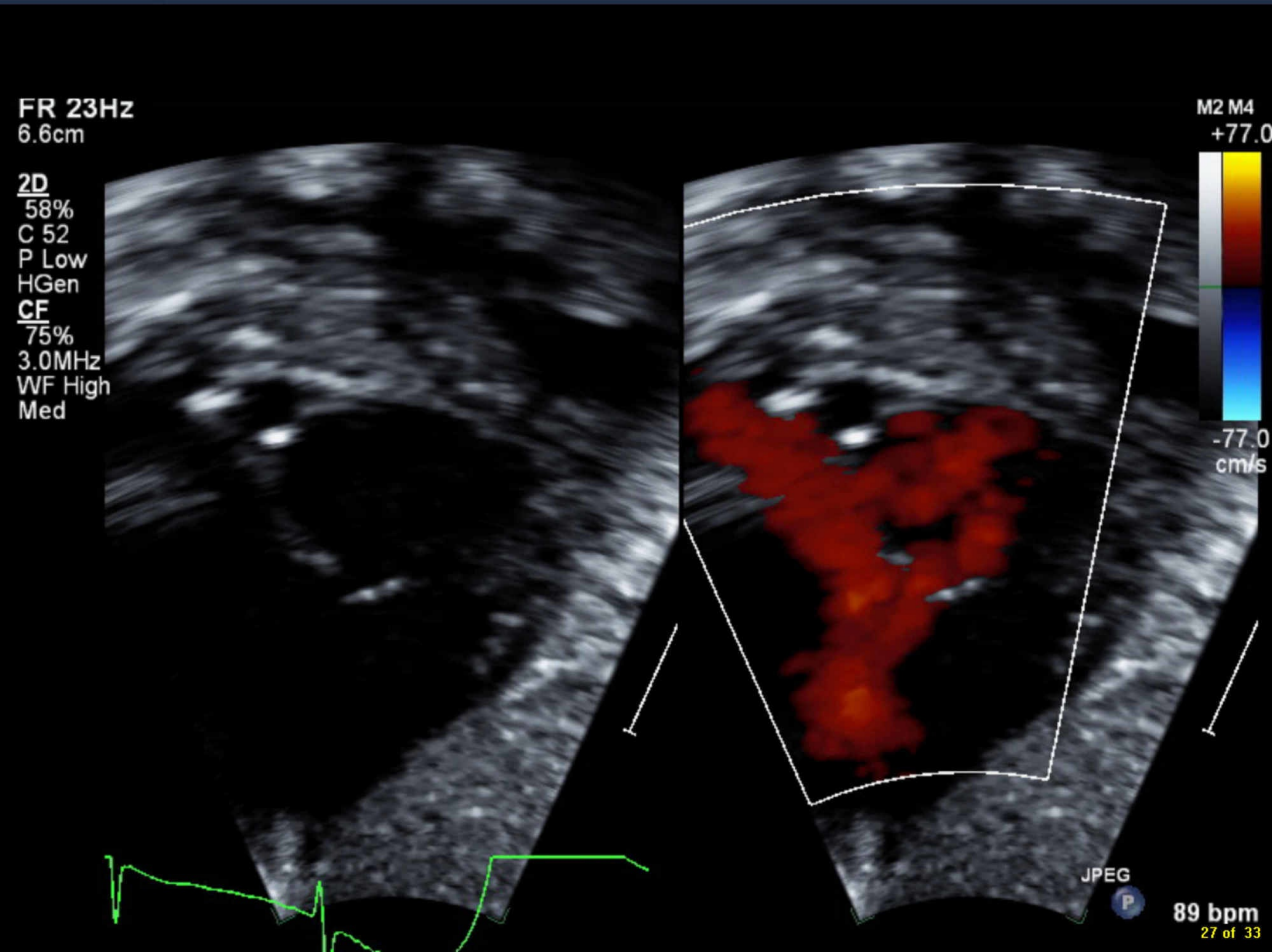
Echocardiography showing patent foramen ovale
